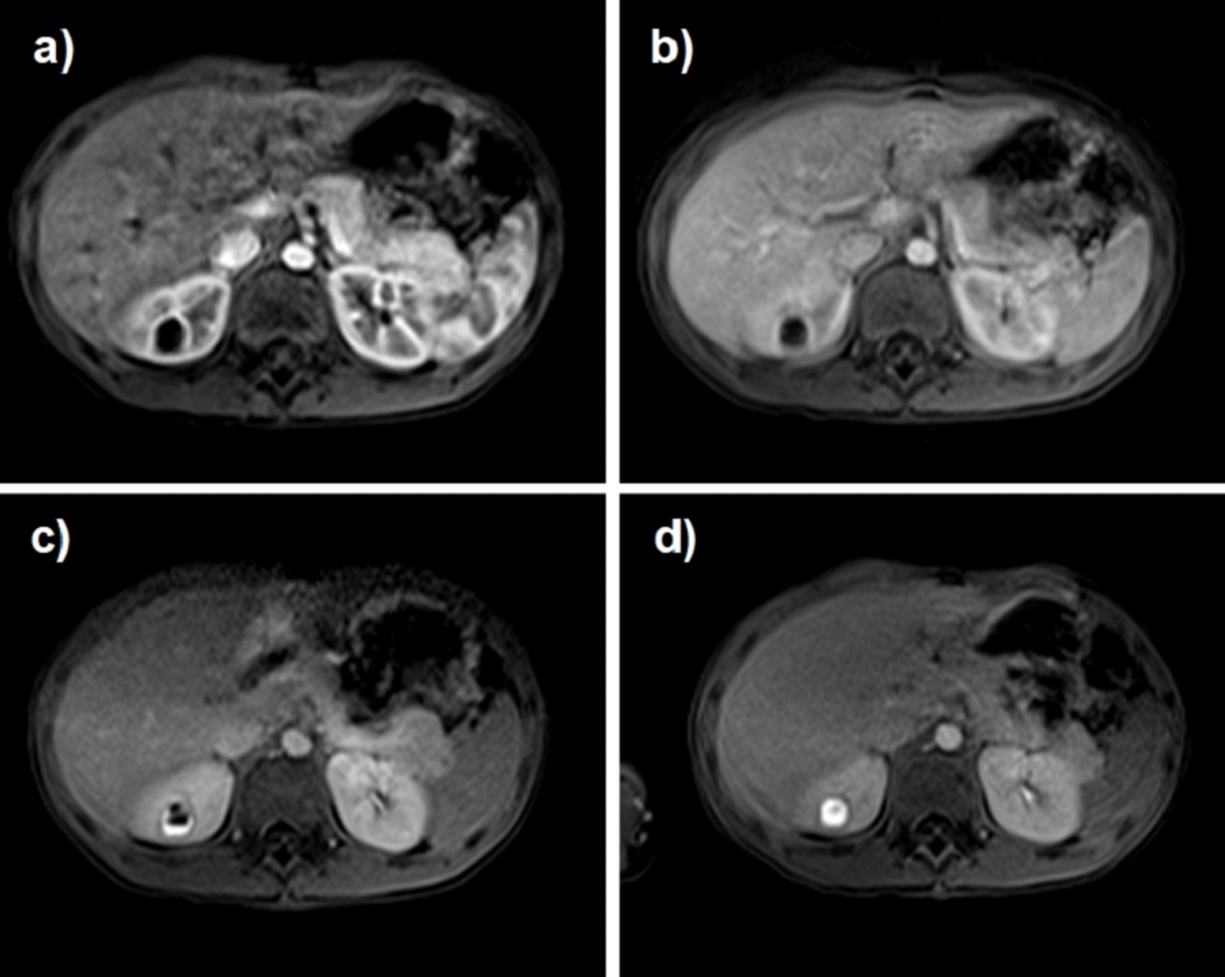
Magnetic resonance imaging (T1) showing a nodular lesion with peripheral enhancement in the arterial phase (a), progressive (b, c) and total enhancement in the excretory phase (d), confirming communication with the collecting system.
